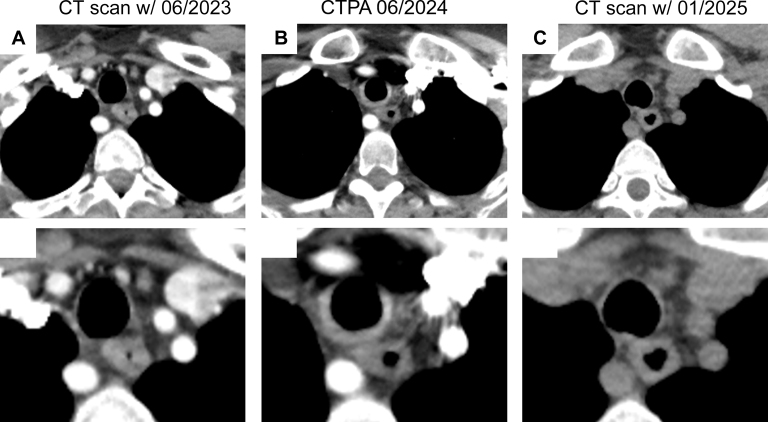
A-C, When compared with the CT thorax scan from 1 y prior (A), the chest CTA with pulmonary artery protocol (B) at the time of admission demonstrated circumferential upper airway wall thickening along the proximal airway. After 6 mo of antifungal treatment, the upper airway thickening resolved on repeat imaging (C). CTA = CT angiography; PA = pulmonary artery; w/ = with; w/o = without.
